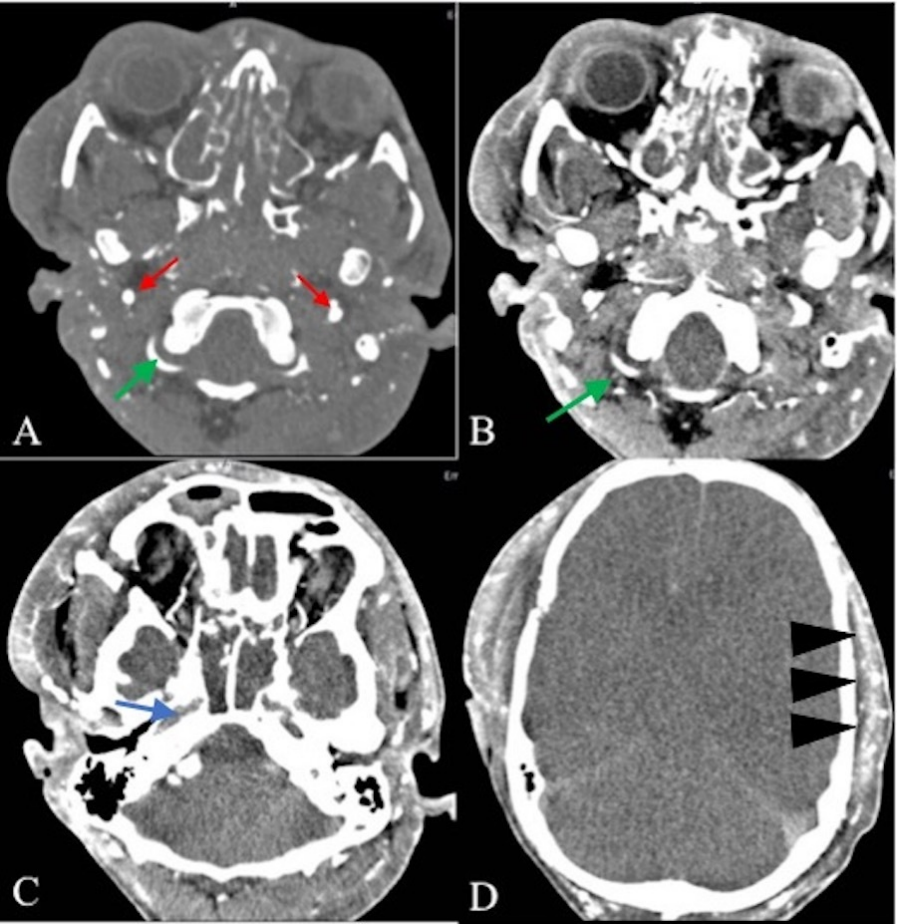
Axial CT angiography of intracranial and extracranial arteries in different levels Consecutive images show flow in the cervical segments of the internal carotid (A, red arrow) and vertebral arteries (A, B, green arrow), which do not continue to the petrous carotid (C, blue arrow) and intradural vertebral arteries (C), and thereafter (D). The extracranial arteries are still opacified (D, arrowheads).
